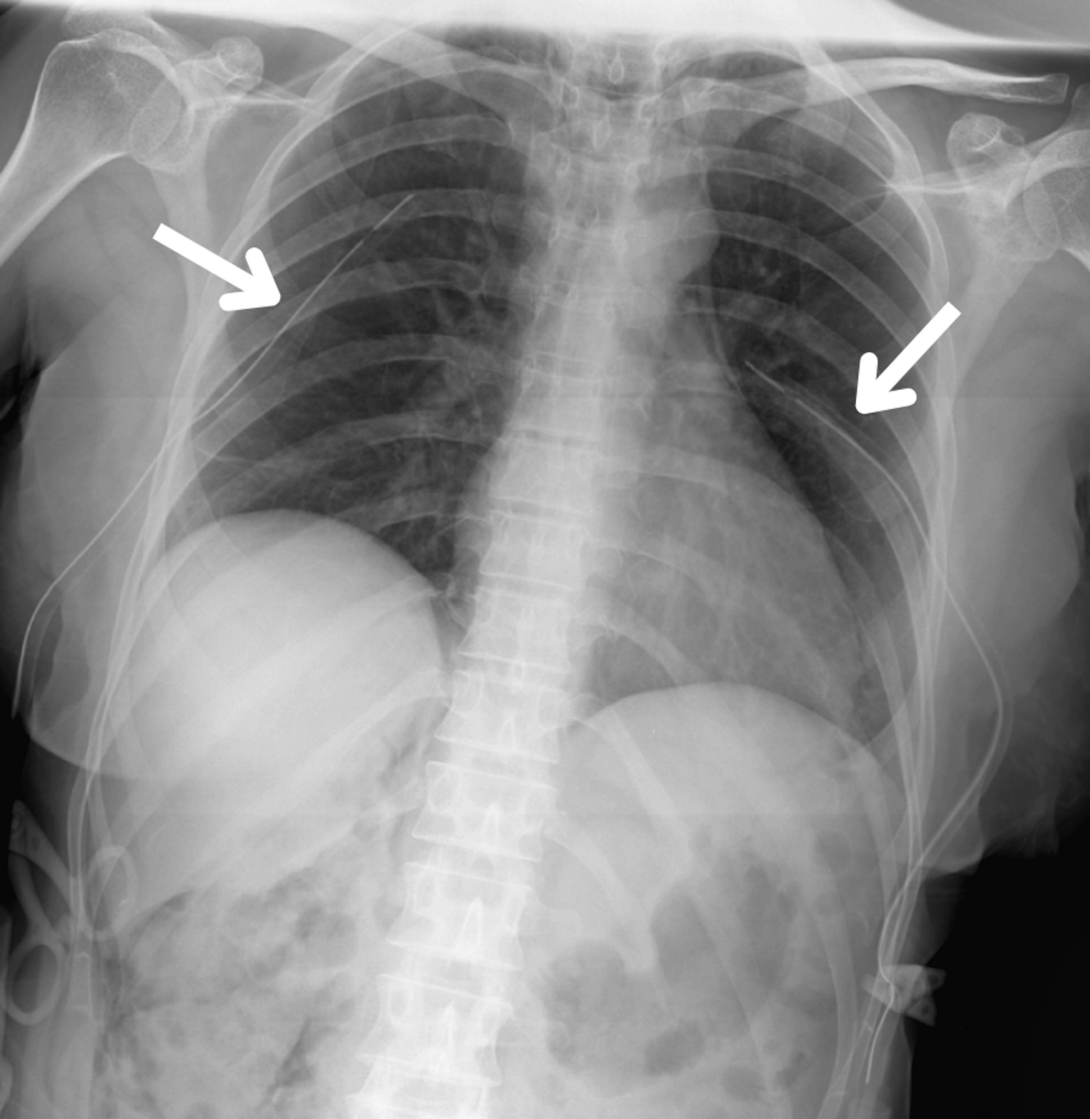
Chest radiography images after bilateral chest tube placement (arrows) with apparent resolution of bilateral pneumothorax.
